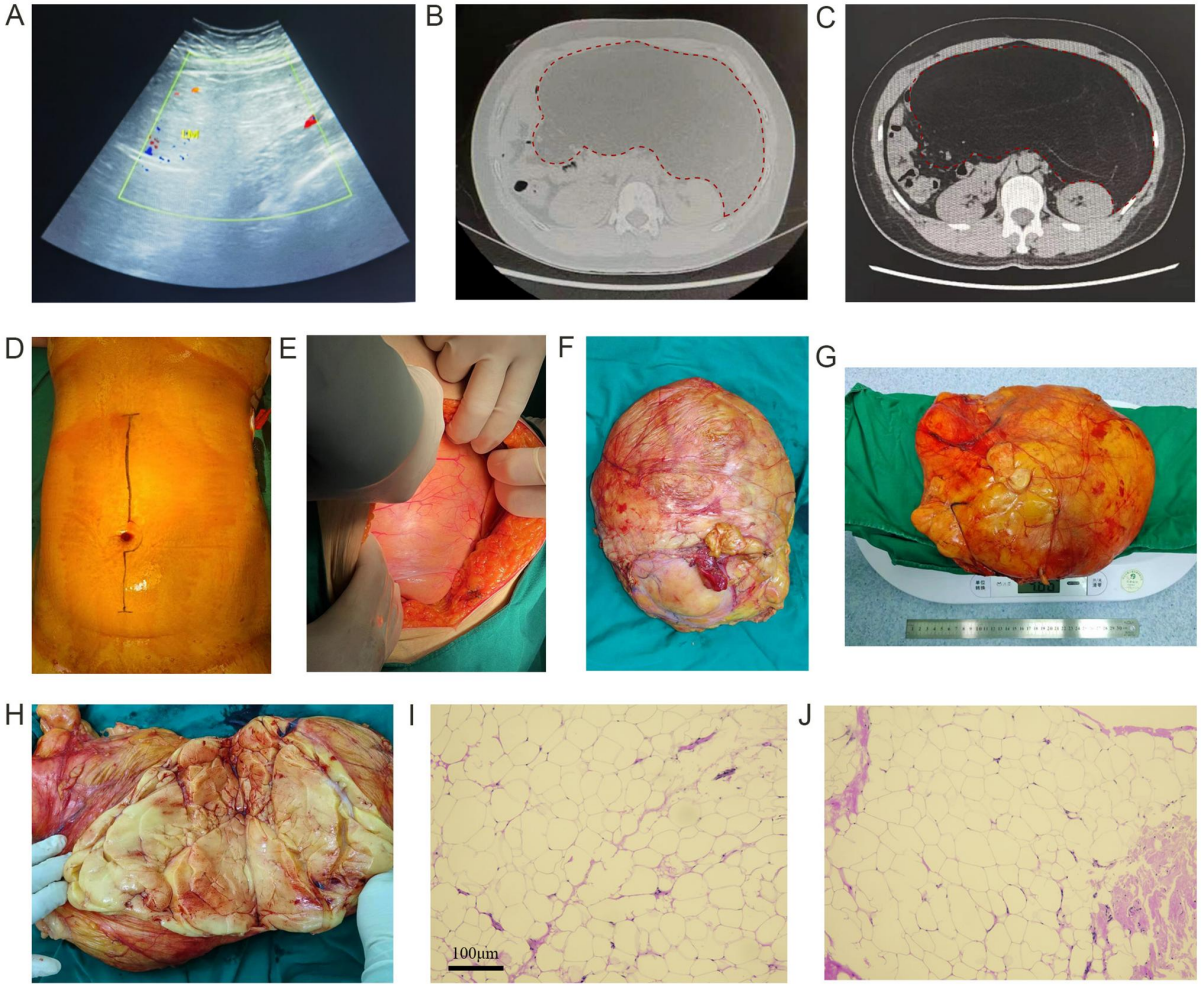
Imaging, intraoperative images, and gross and pathological features of giant lipomas. (A) Patient's B-mode ultrasound; (B), (C) patient's abdominal noncontrast scan with enhanced scan; (D) Preoperative condition of the patient's abdomen, which was measured to be 104 cm; (E) condition of the lipoma seen at the time of intraoperative laparotomy; (F) gross full view of the lipoma (with its coating relatively intact); (G) weighing of the lipoma; (H) longitudinal anatomy of the lipoma.
